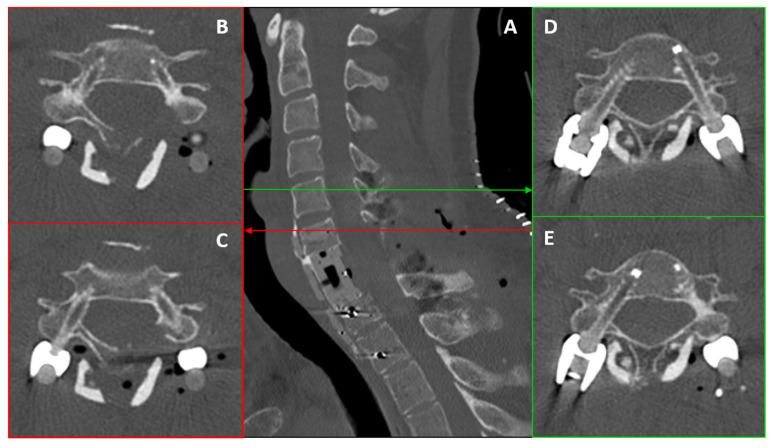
Postoperative Computed Tomography (CT) assessment. (A) Central midsagittal reconstruction showing anterior column restoration. Note the radiolucency of the CFR-PEEK cage and anterior plate, identified by radiopaque markers, allowing artifact-minimizing visualization of the canal and fusion bed. (B,C) Axial views on the left side of the panel, corresponding to C6. (D,E) Axial views on the right side of the panel, corresponding to C5. In these postoperative CT images, orientation follows standard radiologic convention (patient’s right displayed on the left) and is therefore mirrored relative to the ipsilateral orientation used in Figure 4. The axial images confirm the planned trajectories: left-sided screws follow a standard path, whereas right-sided screws at both levels (particularly evident at C5 in (D,E), also appreciable at C6 in (B,C)) exhibit the intentional, small, controlled medial cortical breach. This trajectory accommodated the 4.5 mm screw within hypoplastic pedicles, preserving the lateral wall and protecting the right vertebral artery. For clarity, the corresponding intraoperative planning images for the same slices are shown in Figure 4A,B and are superimposable on the postoperative CT; screw contours, pedicle cortices, and the relationship to the vertebral artery are indicated for direct planned-versus-achieved comparison.
